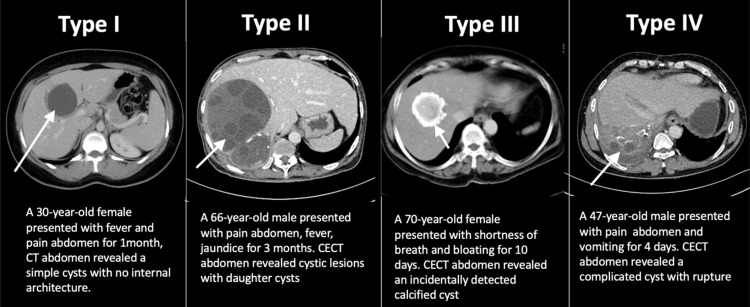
Types of hepatic hydatid cysts with imaging features CECT: contrast-enhanced computed tomography
